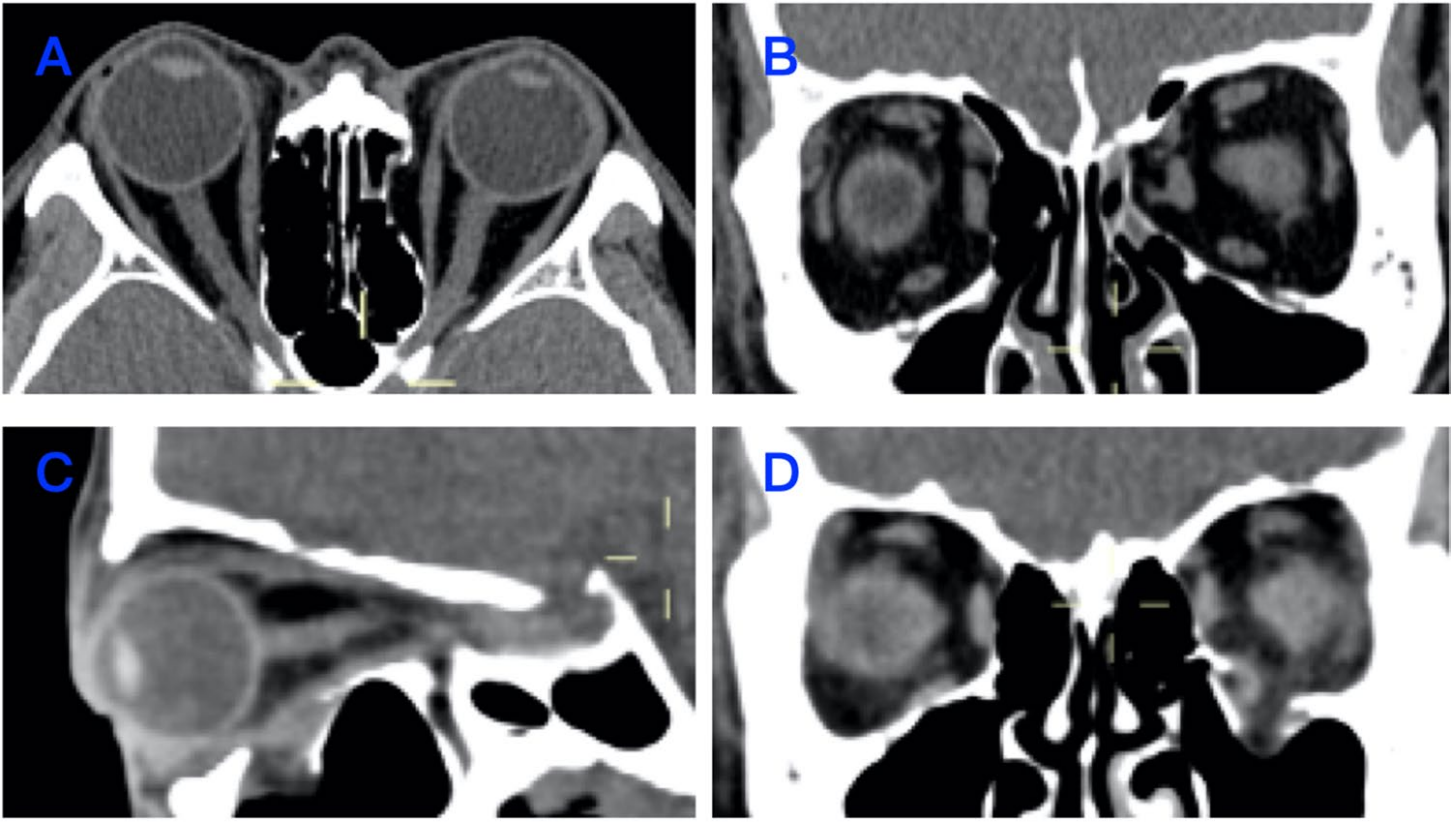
Examples of medial wall and inferior wall orbital fractures. (A) Axial orbital CT for MWF. (B) Coronal orbital CT for MWF. (C) Sagittal orbital CT for IWF. (D) Coronal orbital CT for IWF.
